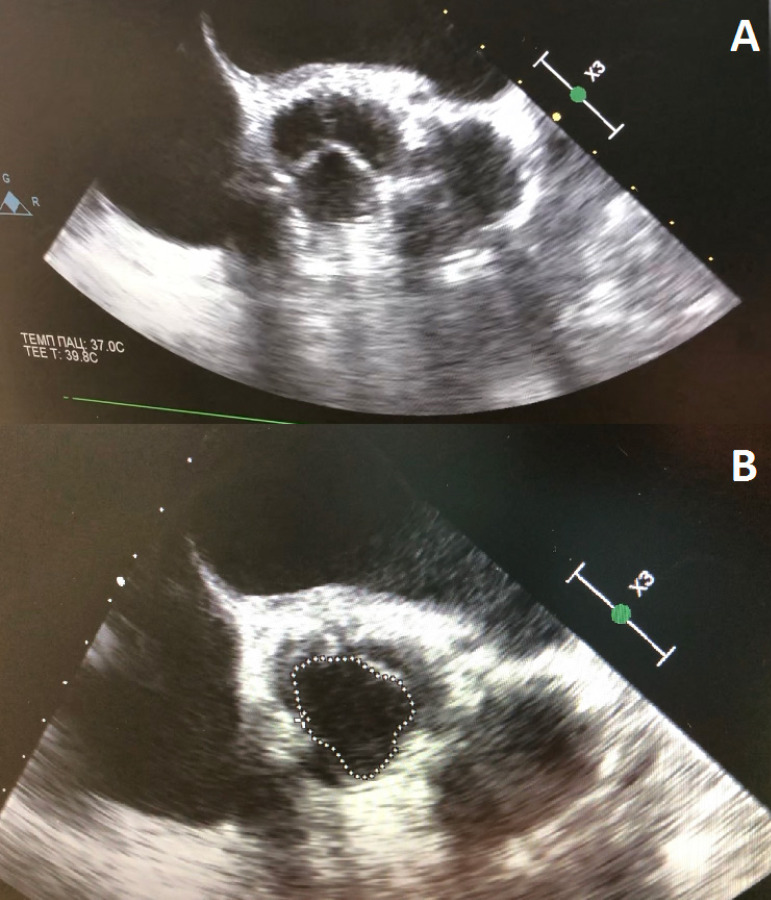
Postoperative echocardiographic views in diastole (A) and systole (B).
